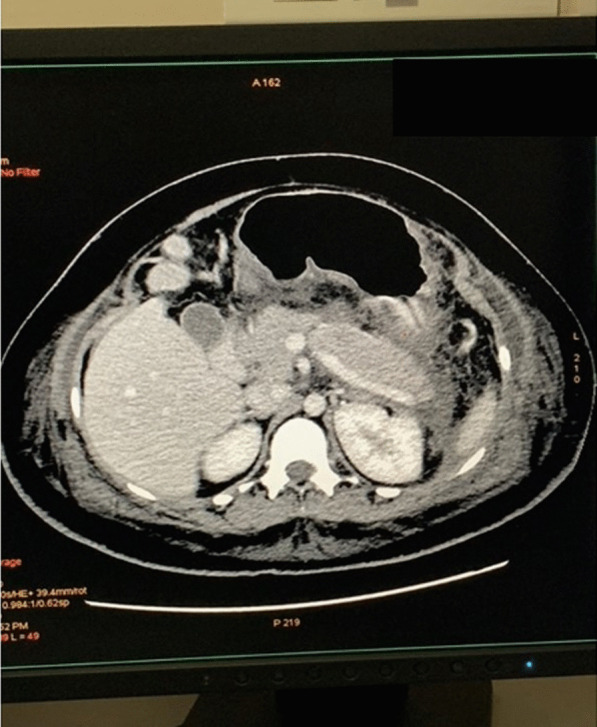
Computed tomography abdomen revealing bulky pancreas with mild enhancement, marked peripancreatic fat stranding with inflammation. (patient’s details shadowed)
